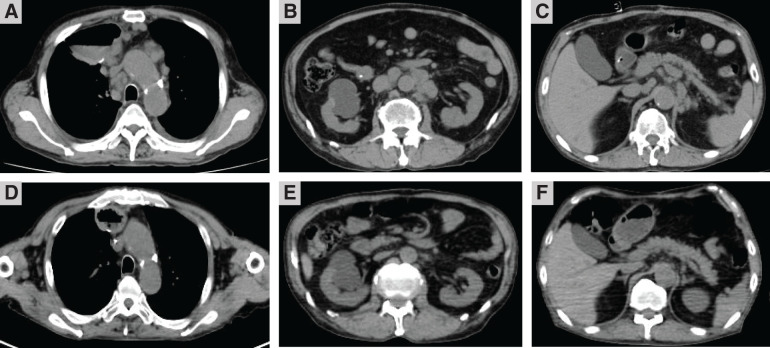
CT findings. CT shows extensive lymph node metastases involving the mediastinum, abdomen, and para-aortic area before immunochemotherapy (A–C), and CR after immunochemotherapy (D–F). CR, complete response
